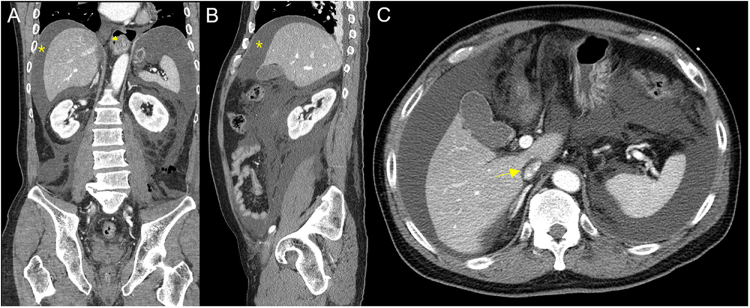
The computed tomography (CT) scan of the abdomen at the time in the emergency department. (a) Coronal; (b) sagittal; (c) axial cut. The coronal and sagittal cuts showed massive intra-abdominal fluid (yellow asterisk) infiltrating the left retroperitoneal space, compressing the kidney vertically and displacing the liver medially and posteriorly. In the coronal view, high intra-abdominal pressure caused the stomach to migrate upward, resulting in hiatal herniation (yellow arrowhead). The axial cut revealed a remarkable finding in the portal vein, which was narrowed by high intra-abdominal pressure (yellow arrows).
